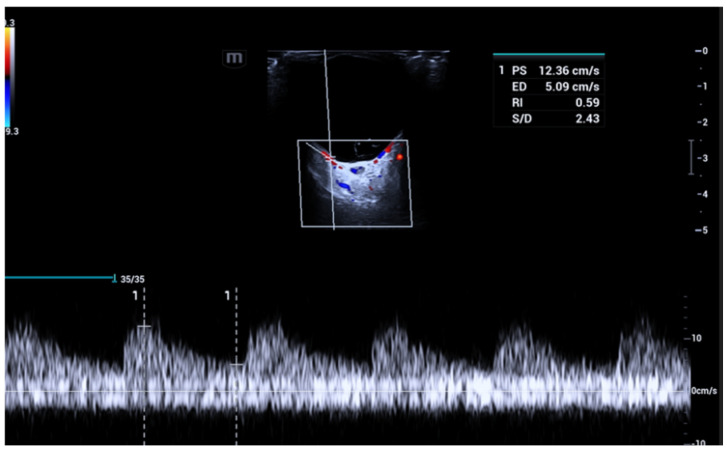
Long posterior ciliary arteries (LPCAs) are depicted in red, coursing parallel to the ocular globe and are often perpendicular to the ultrasound beam; therefore, their Doppler signal may be more difficult to record.
